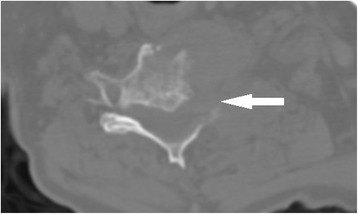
Axial CT demonstrating destructive mass C6 left vertebral body and transverse process
